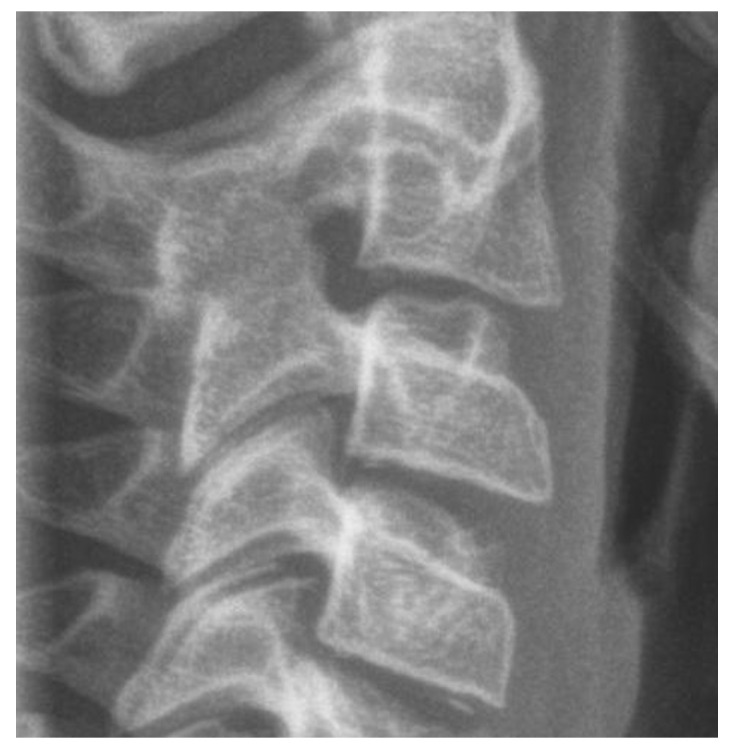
Cervical vertebrae radiograph—rectangular horizontal shape.
